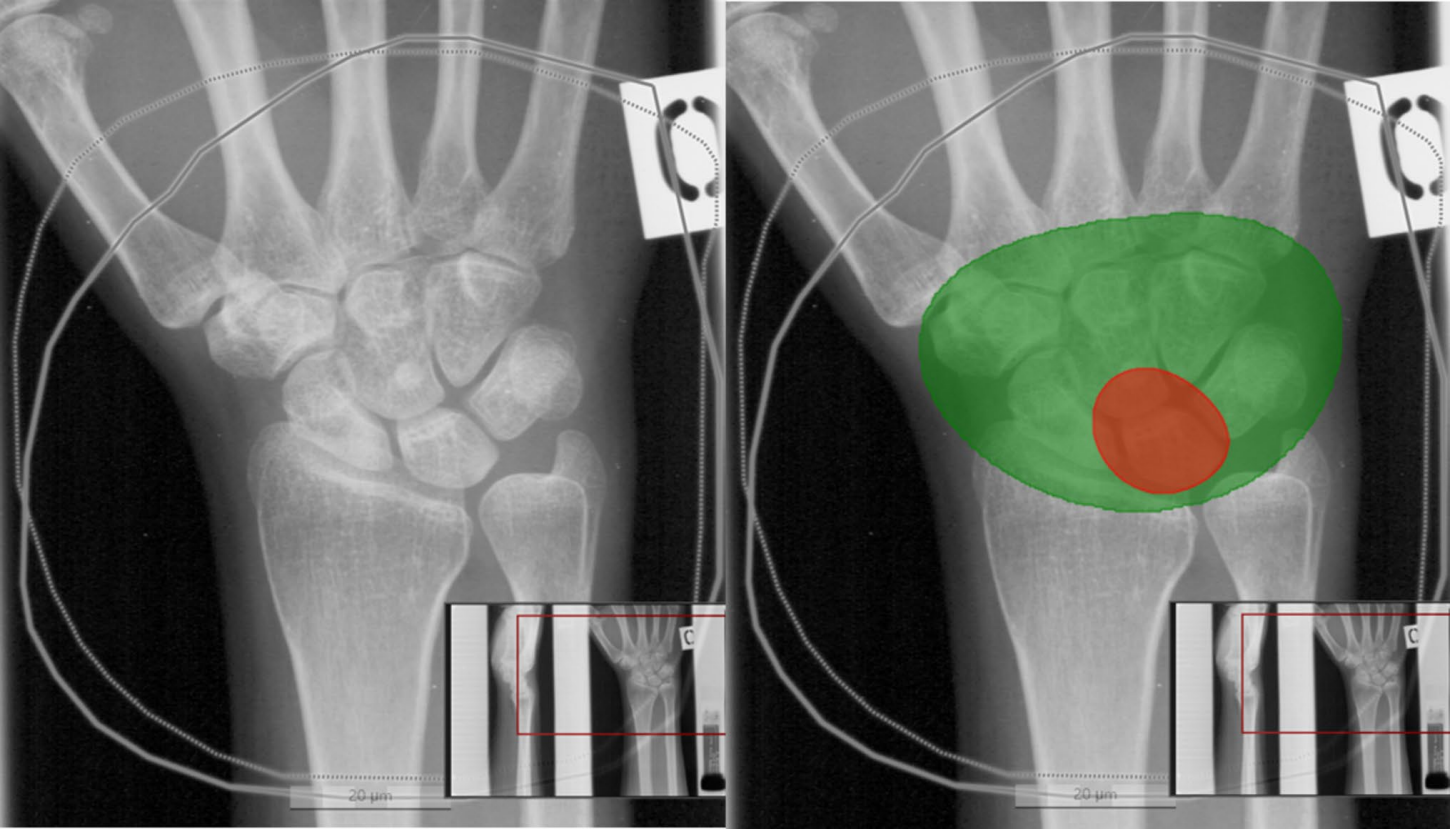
Figure indicates how the DL model recognizes avascular necrosis of lunate stage I from the radiograph that cannot be recognized by human assessment
